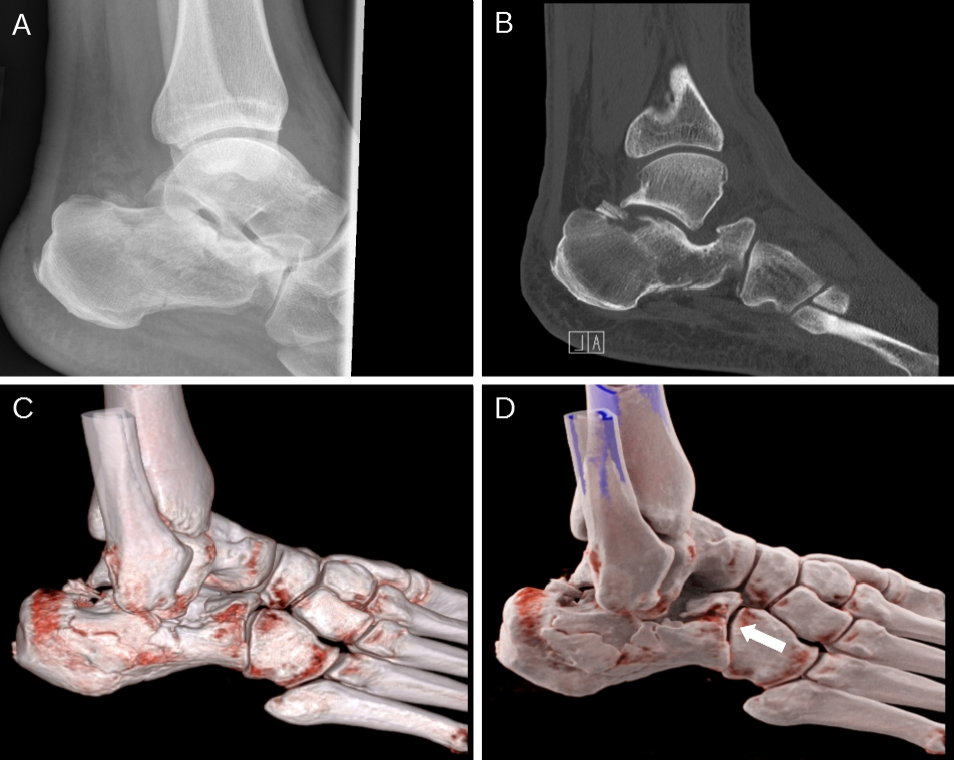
Fig. 1 A 58-year-old male patient who had suffered ankle distorsion after falling down a flight of stairs. Conventional (A) and sagittal MPR images of the CT data set (B) are displayed. While the fracture of the anterior process of the calcaneus is difficult to delineate in the VRT image (C), CR reconstructions (D) clearly demonstrate the fracture lines (white arrow).
